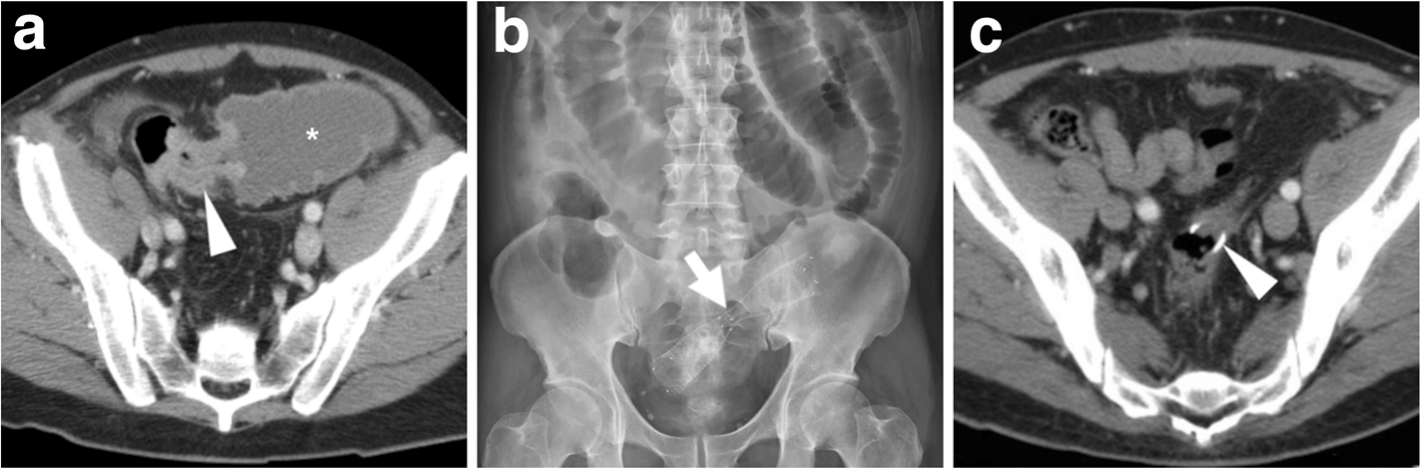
Delayed relief of LBO during bridge-to-surgery stenting of CRC. Initial CT (a) shows luminal dilatation (*) above a stricturing tumour of the sigmoid colon (arrowhead, note shouldering). Early radiograph (b) shows persistent colonic dilatation despite correct placement of the SEMS (thick arrow) at the site of CRC. The patient experienced slow clinical and radiographic improvement and had neoadjuvant chemotherapy followed by resection of pT3N2 cancer with primary anastomosis. Note suture on early postoperative CT (c) performed to exclude anastomotic dehiscence
